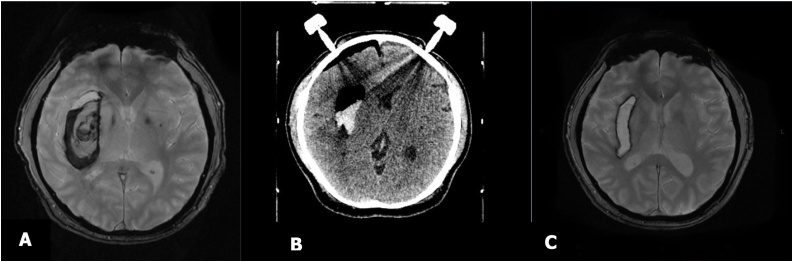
A. MRI image performed a few hour after onset, B. Post-operative CT Scan after stereotactic surgery immediately, and C. MRI image 2 months after surgery.
